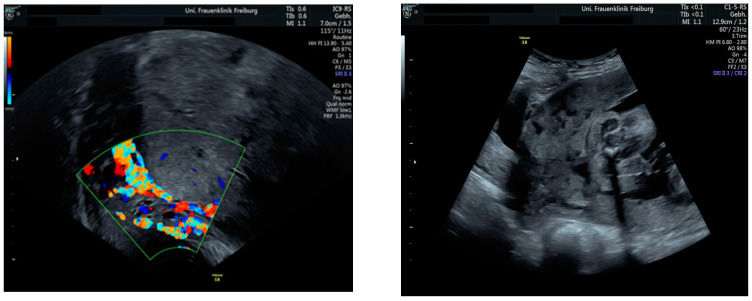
Increased placental blood flow on 2D ultrasound. Red color represents increased placental blood flow moving to the transducer; blue color represents placental blood flow moving leaving away from the transducer.
